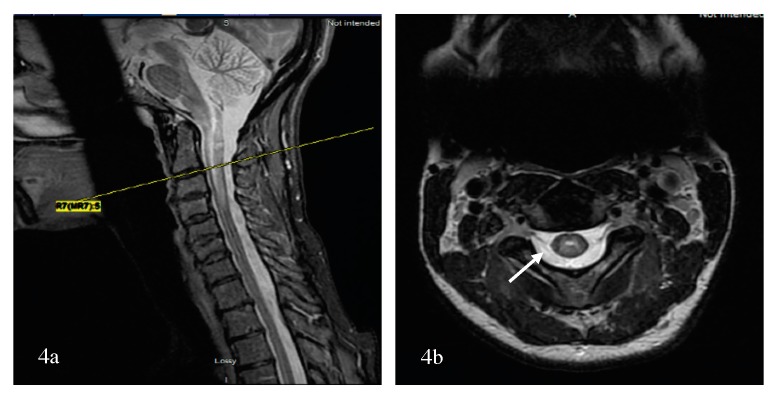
55-year-old African-American female, with AQP4-NMOSD; sagittal STIR cervical MRI demonstrates simultaneous linear lesion and LETM from the medulla to C4 (4a) seen at the center of the cord on axial T2 (4b).
